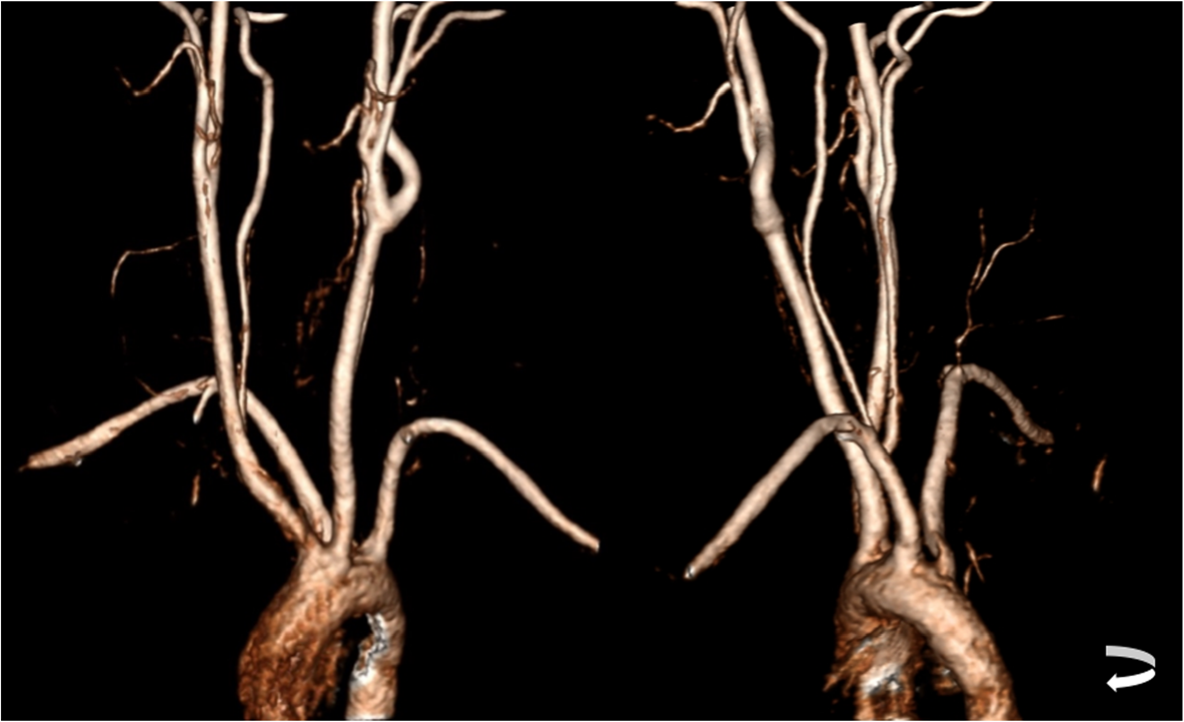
Three-dimensional reconstruction of a CT angiography of the supra-aortic trunks of a 49-year-old patient with Down syndrome depicting an aberrant right subclavian artery
